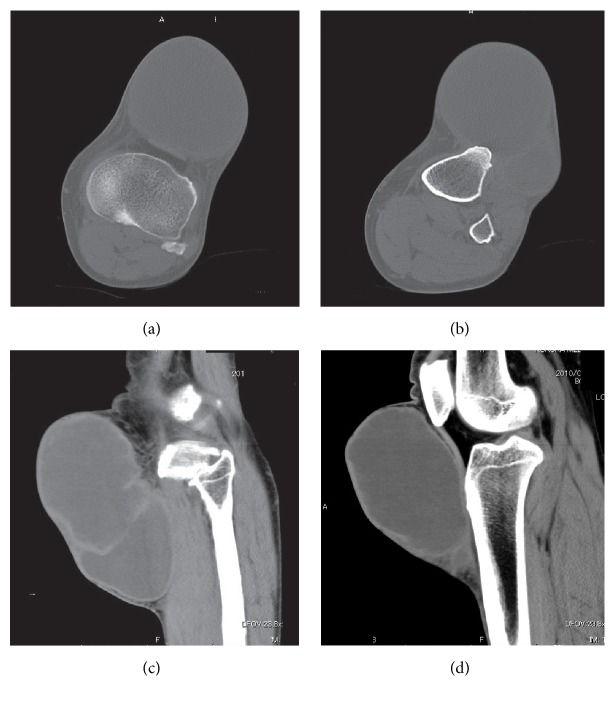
61-year-old male with a subcutaneous chronic expanding hematoma in the knee region. Computed tomography shows a cystic lesion with a pseudocapsule and a septum. Subcutaneous fat tissue is not observed between the lesion and the overlying skin ((a-b) axial bone window; (c-d) sagittal soft-tissue window).
